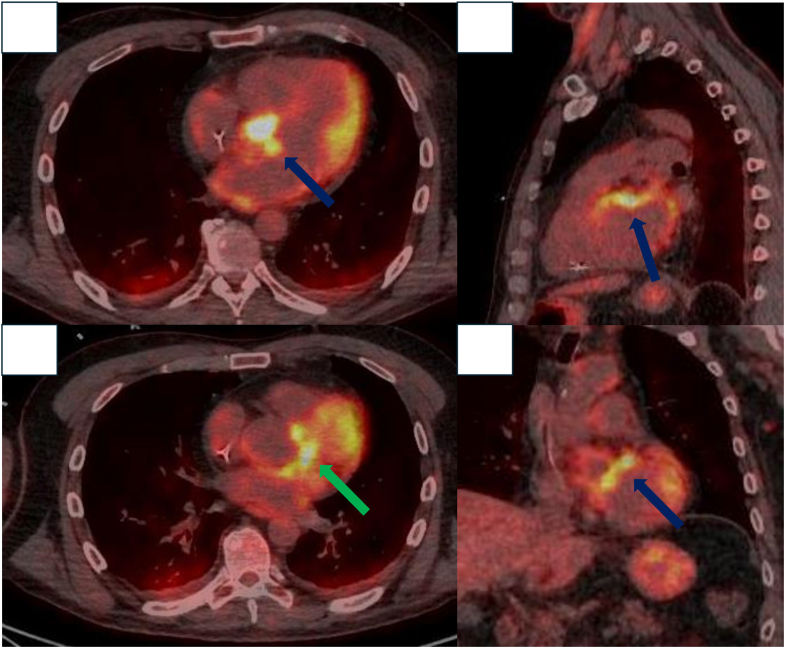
^18^F-FDG PET-CT Crescent-shaped intramural inflammatory uptake involving the aortic root, with involvement of the aorto-mitral junction. ^18^F-FDG PET-CT = fluorine-18 fluorodeoxyglucose positron emission tomography-computed tomography.
